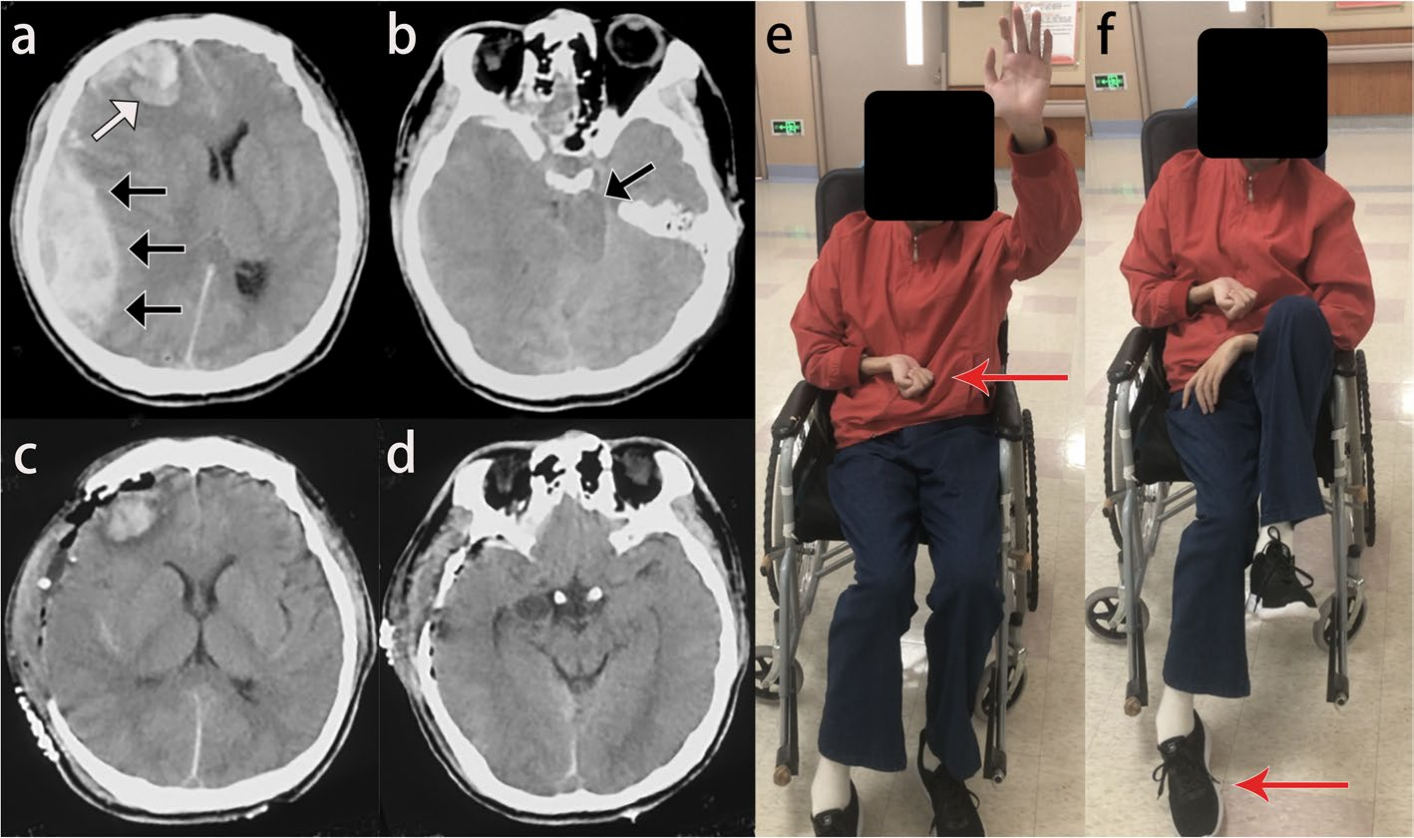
a Preoperative computed tomography (CT) scan revealed a right epidural hematoma (black arrows) and frontal lobe contusion (white arrow) with significant right-to-left midline shift. b A descending transtentorial herniation and total obliteration of all basal cerebrospinal fluid spaces by the displacement of brain can be seen. Note the left-mesencephalon notching against the tentorial edge (black arrow). c, d Postoperative CT scans. e, f The patient’s right hemibody strength was 2/5 (red arrows), indicating a right-sided hemiparesis
